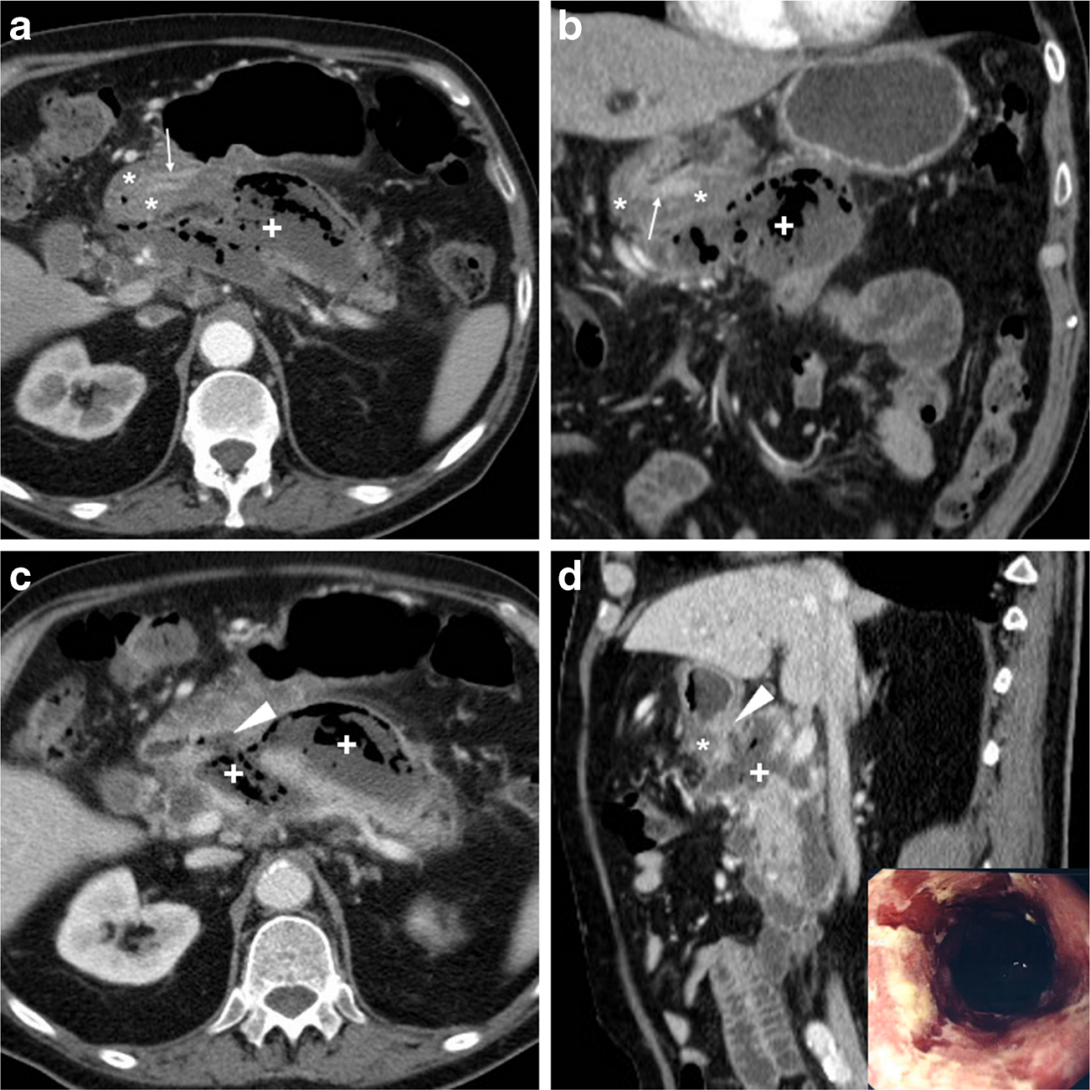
In an 84-year-old male with malaise, appetite loss, epigastric tenderness and protracted low-grade fever, contrast-enhanced CT detected a vast retroperitoneal abscess (+) with some internal gas and residual enhancing pancreatic parenchyma at body and tail, which was indissociable from the distal gastric antrum and pylorus: the latter showed circumferential mural thickening with oedematous submucosa (*) and hyperenhancing mucosa (thin arrows). Additionally, focal communication (arrowheads in c, d) was identified between pancreatic abscess and inflamed digestive tract. The fistulisation site corresponded to endoscopic finding (image in inset d) of large posterior bulbar ulcer. Laparotomic surgery required extensive dissection, confirmed a vast, stinky purulent and necrotic collection, fixed to the stomach and deformed pylorus, and was completed with gastric resection and Billroth-II gastrojejunostomy. Pathology confirmed severe transmural ulcerated peptic gastro-duodenitis, without malignant changes [Adapted from Open Access ref. no [21]]
